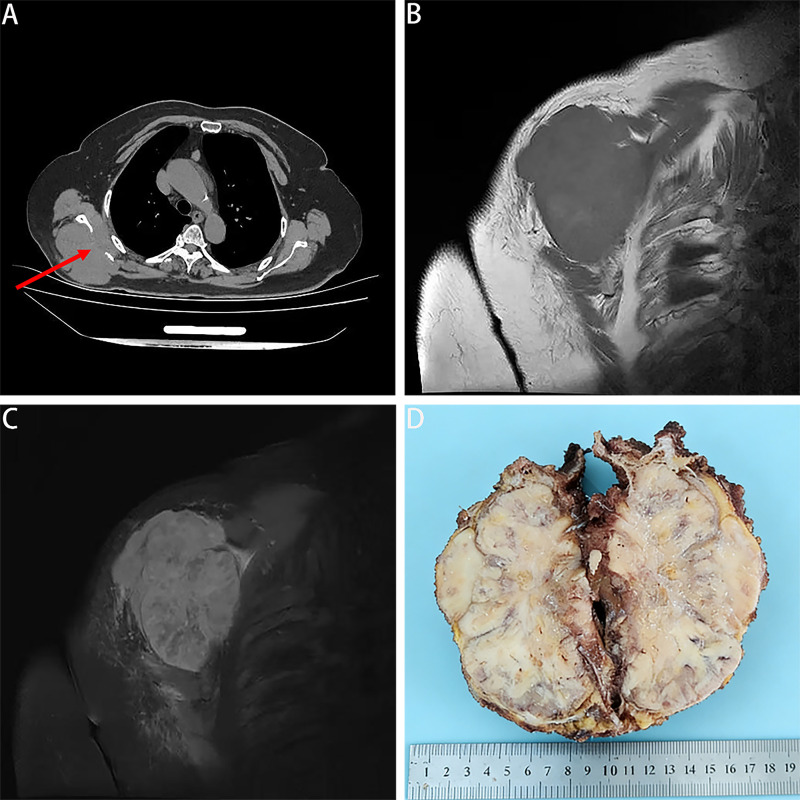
Radiologic and gross features of FRCT. (A) Computed tomography revealed an irregular soft tissue mass lateral to the right scapula, associated with destruction of the underlying bone; (B, C) Magnetic resonance imaging demonstrated an irregular mass lesion with ill-defined margins relative to the adjacent soft tissues. The lesion exhibited heterogeneous signal intensity, with mixed iso-/hypointensity on T1-weighted images (B) and mixed iso-/hyperintensity on T2-weighted images (C) signal intensity, involvement of the adjacent scapula was noted; (D) The cut surface of the tumor was grayish-yellow with irregular but well-demarcated borders.
